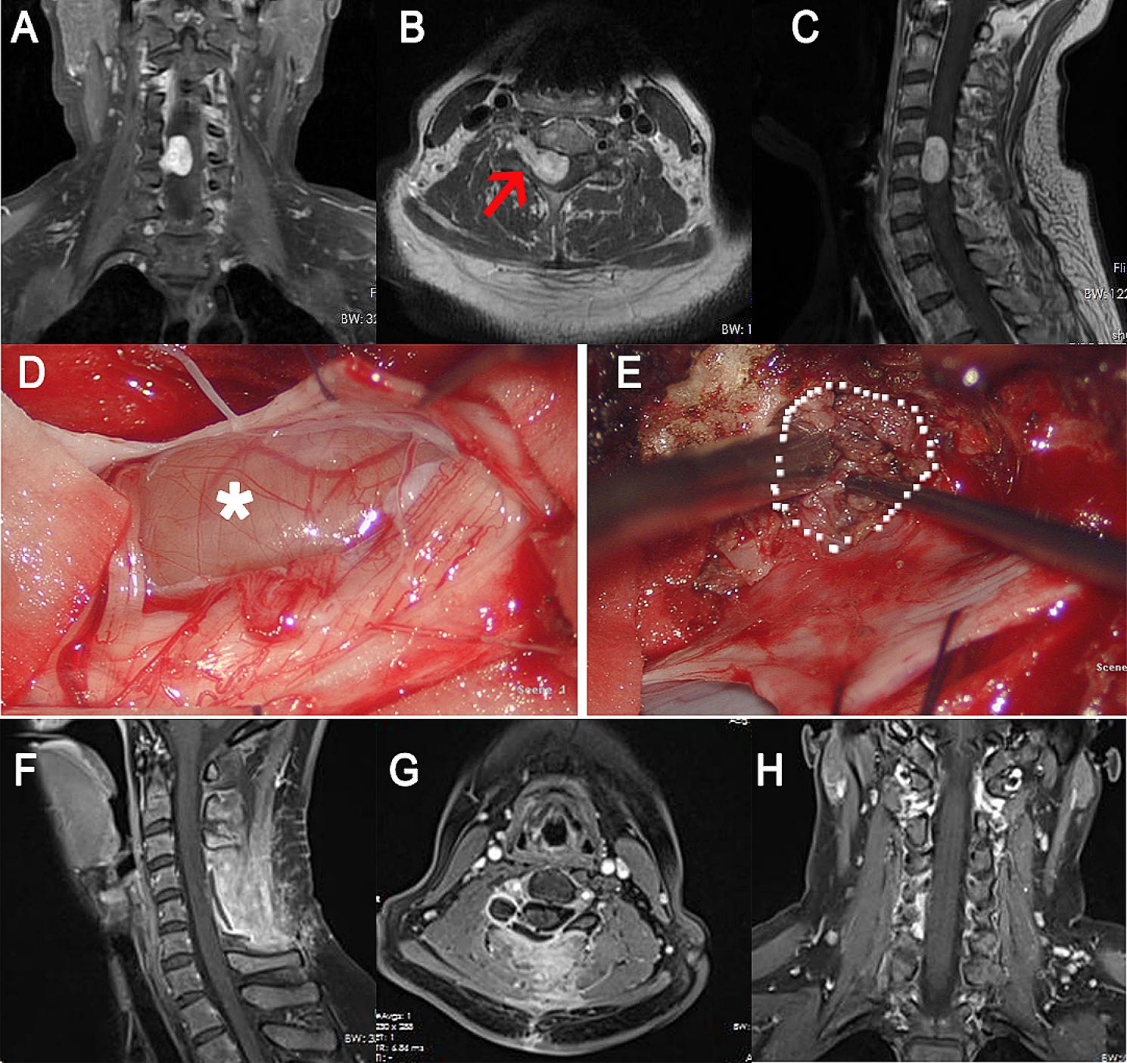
A 40-year-old woman was diagnosed with spinal schwannoma. A, B, and C: preoperative MR images. The tumor (red arrow) was located at the C4-5 level. D and E: images during the operation. The white star indicated the intraspinal portion and the white dot line showed another incision to expose the extraspinal portion. F, G, and H: MRI performed one month after the operation
